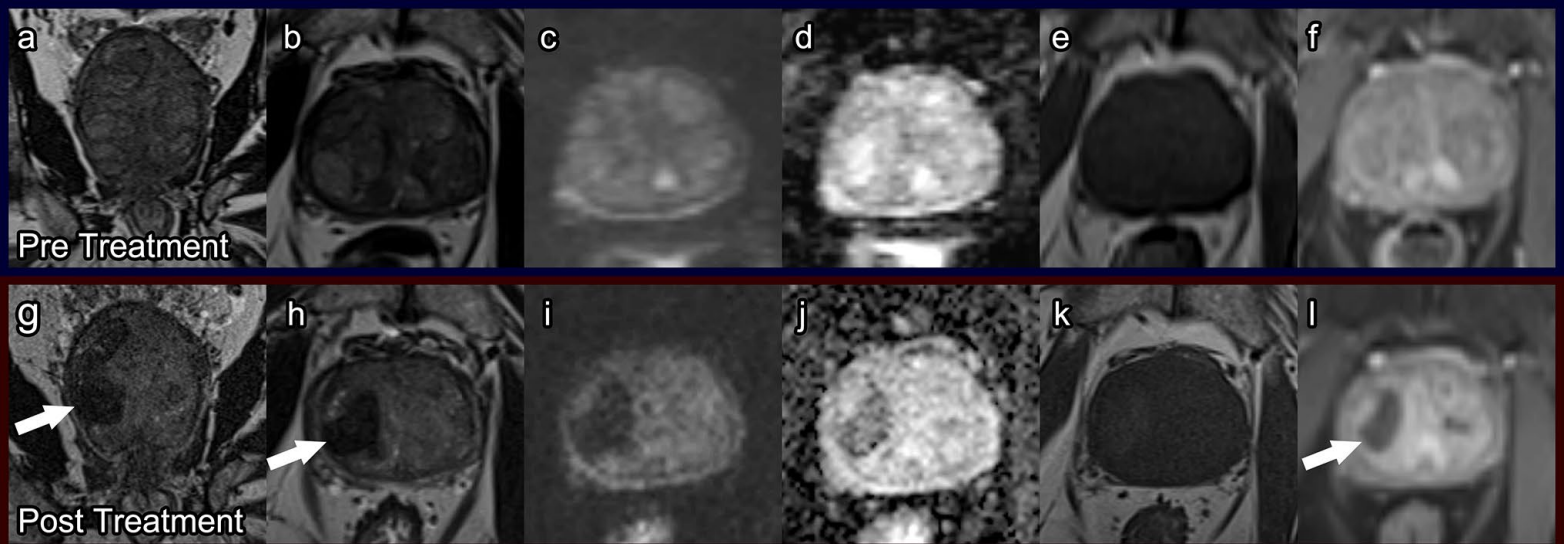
Prostatic artery embolization. Pre-treatment prostate MRI: Coronal T2 (a), axial T2 (b), axial DWI (c), ADC (d), pre-contrast T1 (e), and post-contrast T1 (f) demonstrate prostatomegaly with findings of benign prostatic hyperplasia, without diffusion restriction and with normal enhancement. Post-PAE prostate MRI at 4 months: Coronal T2 (g), axial T2 (h), axial DWI (i), ADC (j), pre-contrast T1 (k), and post-contrast T1 (l) demonstrate T2 dark areas of chronic ischemia with hemosiderin deposition (arrows) no significant diffusion restriction (i), and new hypoenhancement associated with ischemic tissue (arrow, l)
